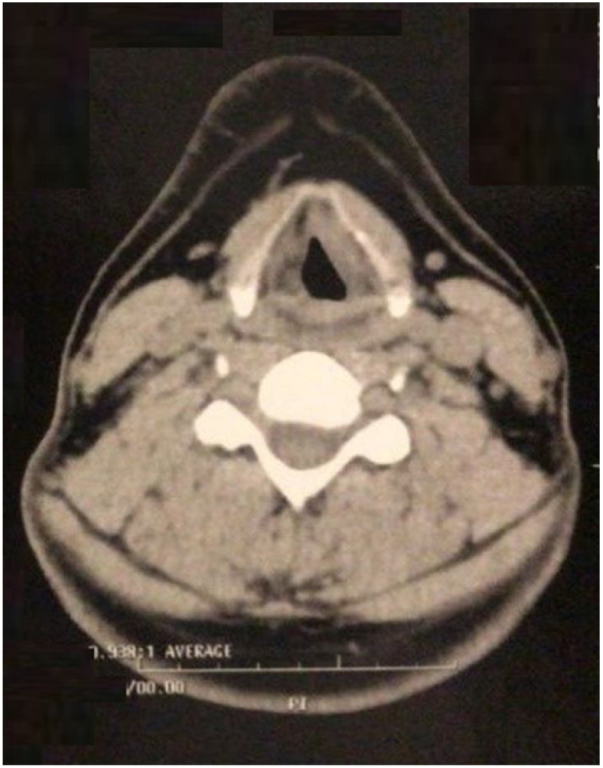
Computed tomography of the neck, axial view. Diffuse mucosal thickening of the larynx, obliteration of the paraglottic fat planes.
